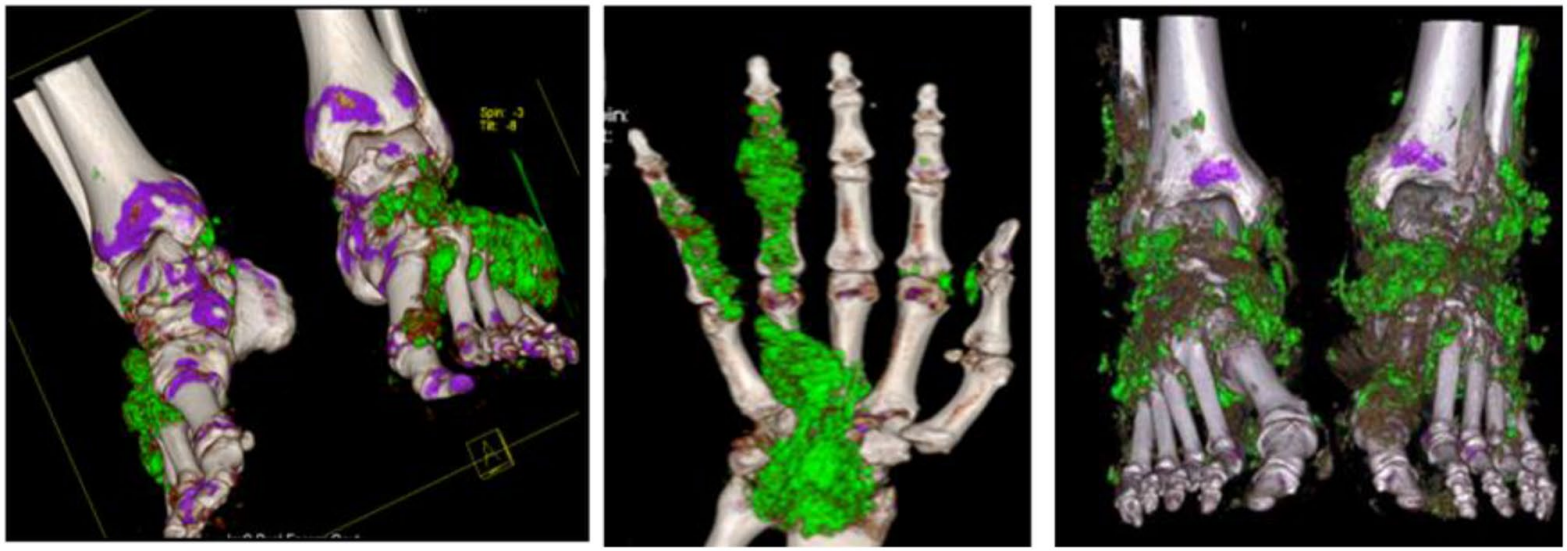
Preoperative three-dimensional CT image
